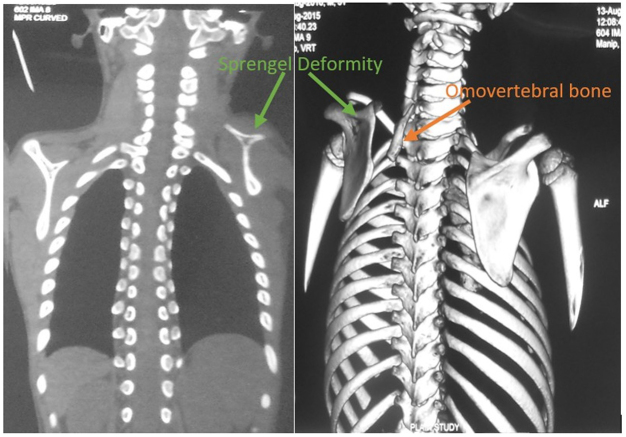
These findings on CT images (coronal and Volume Rendering Technique) are consistent with a left-side elevated scapula (green arrow) with an omovertebral bone (orange arrow) (a bar connecting the scapula with the cervical spine) at the level of the C6 vertebra.
